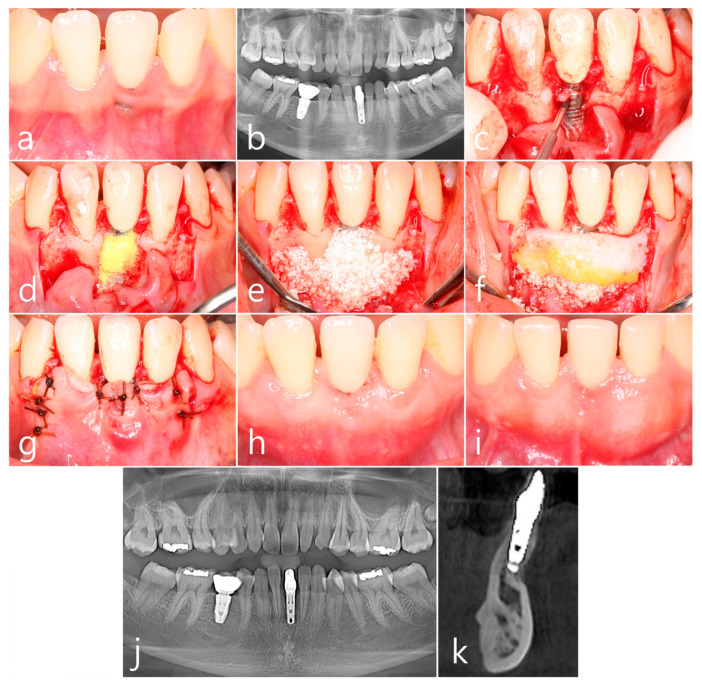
Case 3. (a) Peri-implant soft tissue dehiscence occurred in the labial gingiva 3 years after implant #31 was placed; (b) No crestal bone loss was found in panoramic radiography; (c) A vertical incision was performed in the distal line angle of the adjacent tooth of implant #31, and a crevicular incision was performed to include the buccal interdental papilla. Next, the labial mucoperiosteal flap was reflected. The implant surface was exposed on the labial surface of implant #31. Surface decontamination was performed using a rotary titanium brush; (d) Additionally, chemical detoxification was performed with Tc HCl. Sufficient saline irrigation was performed; (e) A synthetic bone graft substitute was used for bone grafting; (f) An SCTG obtained from the left palatal surface was fixed with 4-0 catgut on the bone graft; (g) A mucoperiosteal flap was also closed with a 4-0 catgut; (h) In the clinical finding 1 month after the procedure, the dehiscence of the peri-implant mucosa disappeared, and the esthetics were restored; (i) In the clinical findings 4 years after the procedure, the thickness of the peri-implant mucosa was further increased; (j) No change in crestal bone was observed in panoramic radiography taken 4 years after the procedure; (k) Labially augmented and corticalized bone of implant #31 was observed in cross-sectional images of CBCT taken 4 years after surgery.
